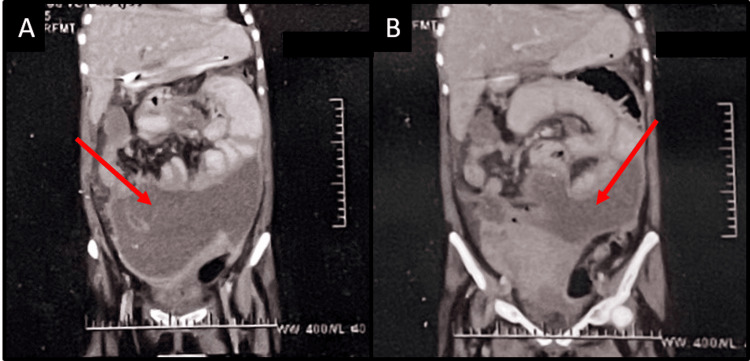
The CT of the abdomen (A and B) shows a large, peripherally enhanced collection intraabdominally anterior to the uterus and peritoneal thickening suggestive of peritonitis.
